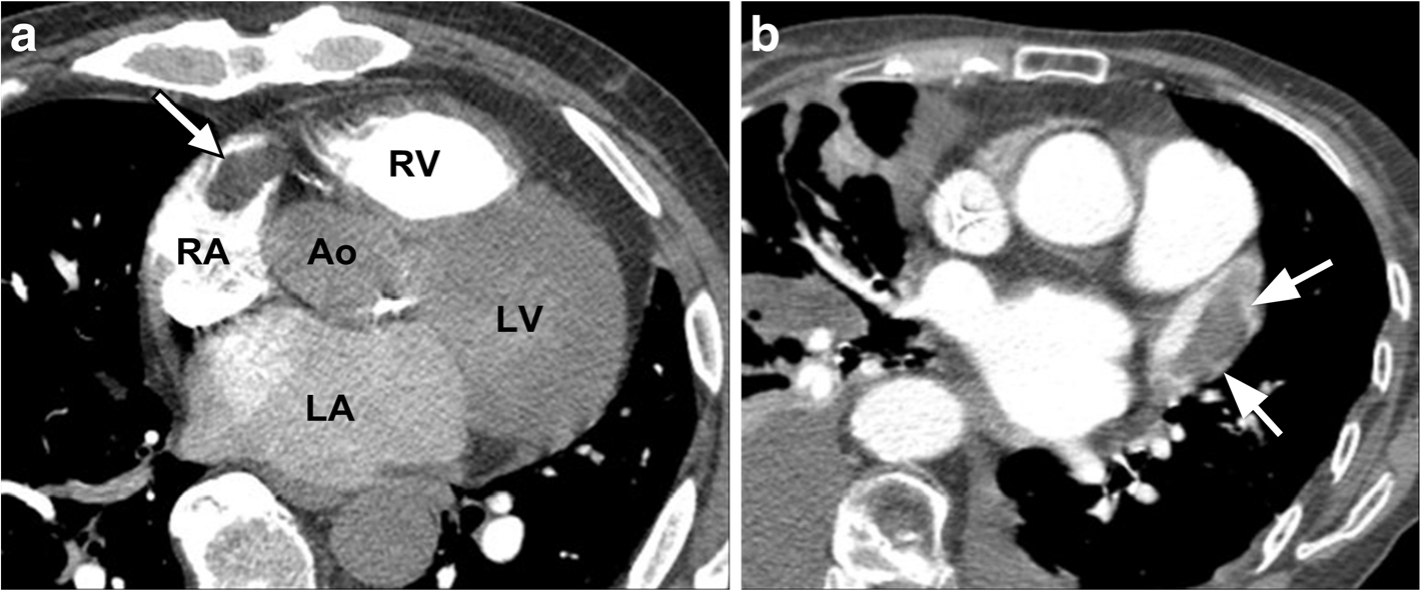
a Axial CT image demonstrating thrombus (black arrow) in the right atrium in a 73-year-old patient who had CT to evaluate for pulmonary embolus. b Axial CT image demonstrates thrombus within the appendage of the left atrium (white arrows) of another patient who had atrial fibrillation who was being evaluated for pleural effusions
